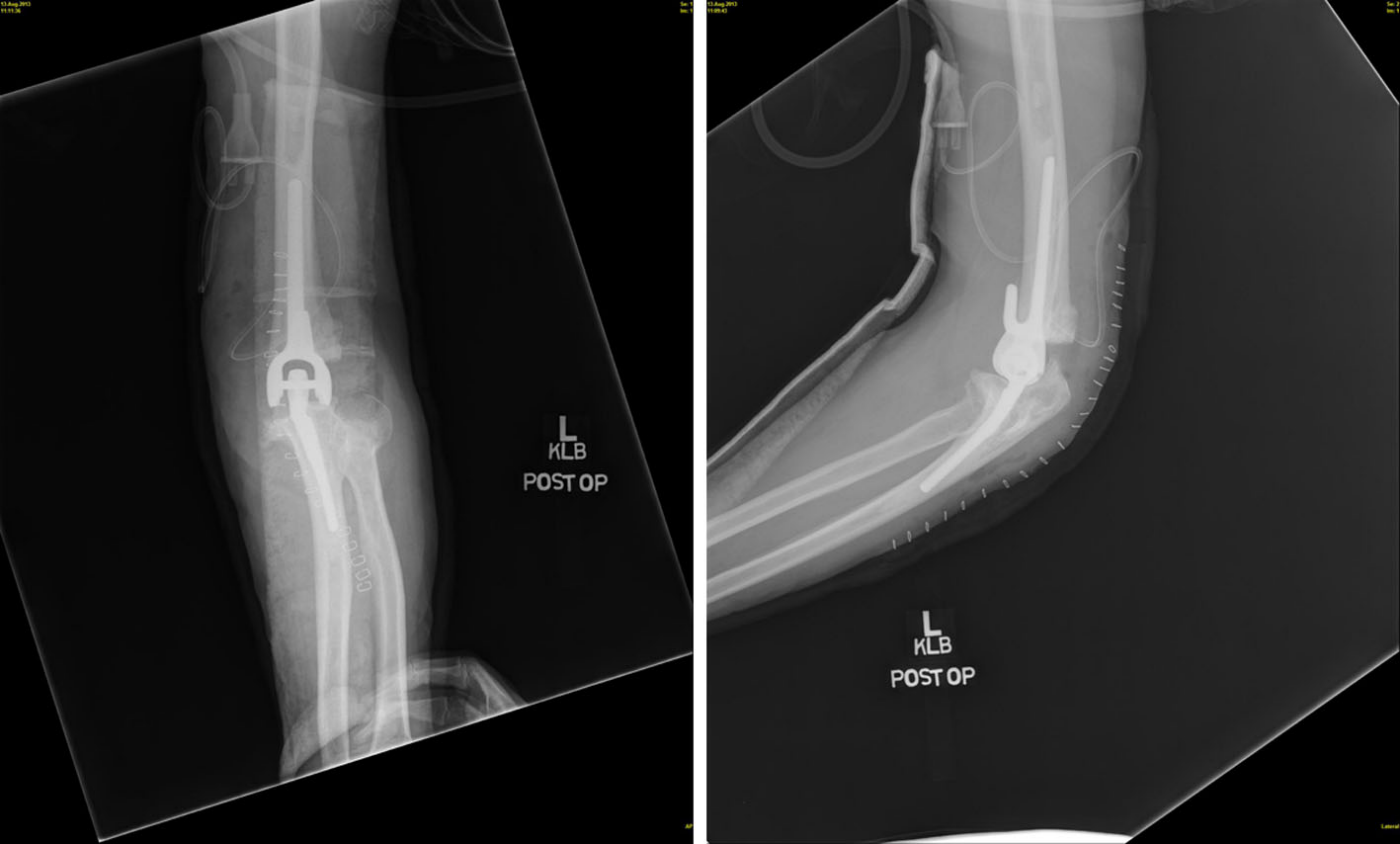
Nexel total elbow; this prosthesis also has a humeral anterior flange. As a result of the ticker bearing, there is more space between the two components on the X-ray compared with the Coonrad/Murray total elbow. The joint surface of the humeral component of the Nexel is more circular shaped, in contrast to the Coonrad/Morrey total elbow, best seen on the AP view
